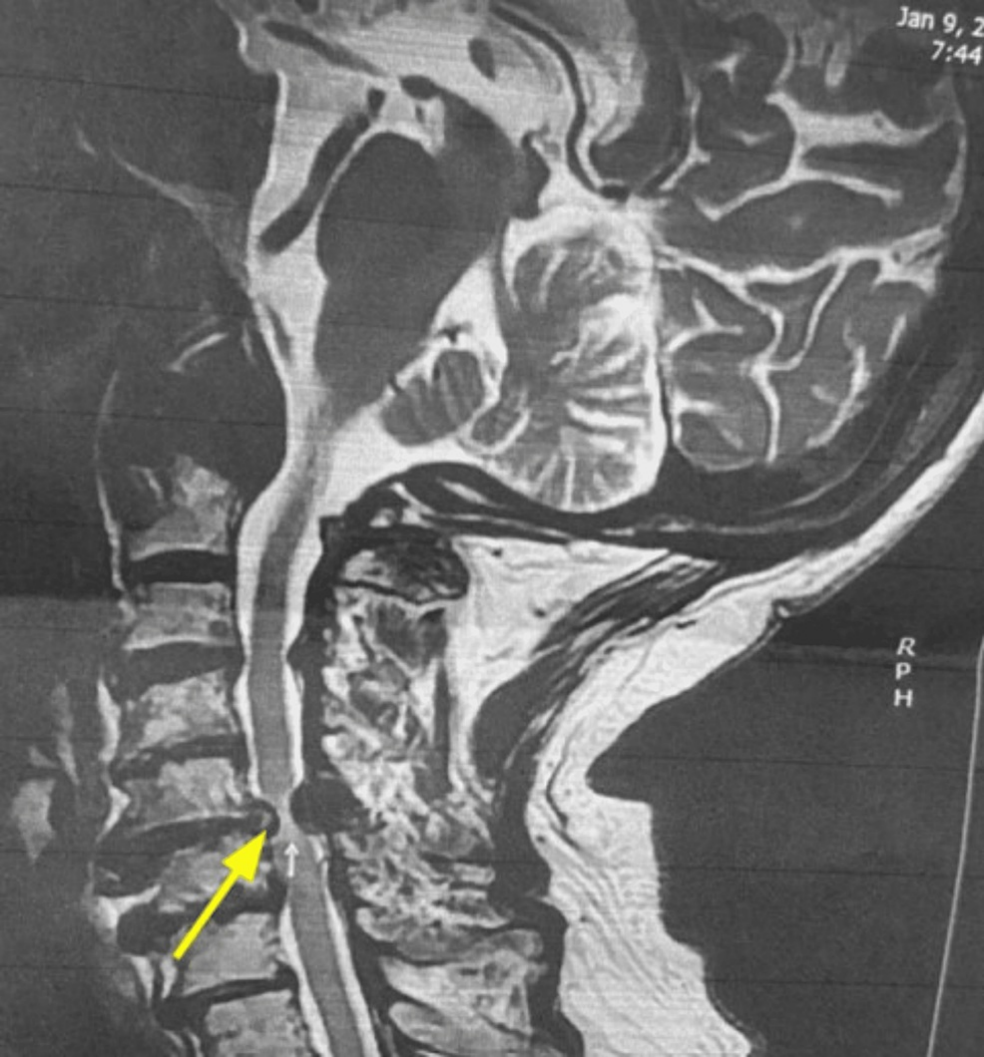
MRI of the cervical spine (axial) showing cervical stenosis, done two years prior to presentation.
